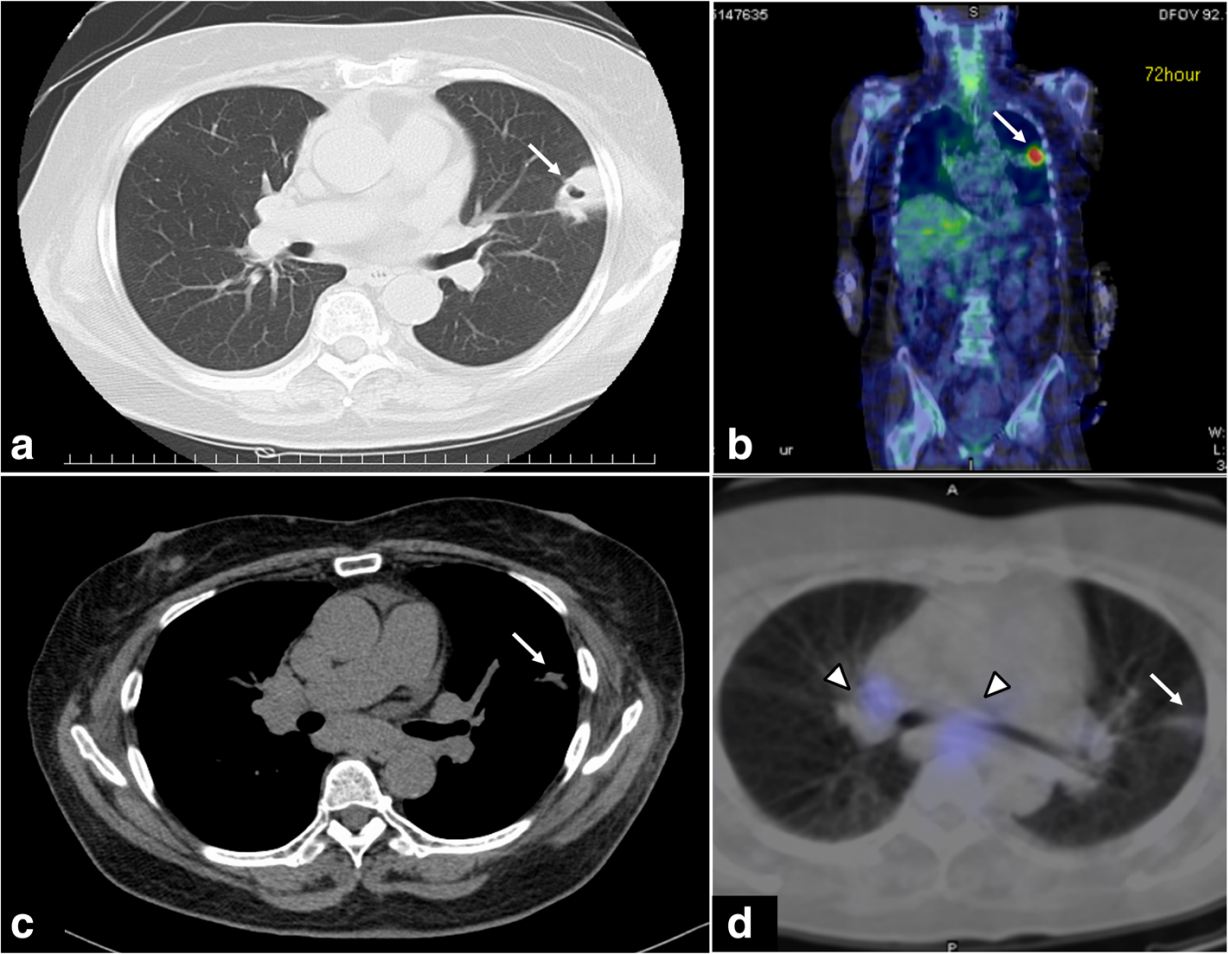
Imaging studies of the lung nodule at the time of hospitalisation (a, b) and 6 months after disease onset (c, d). a. Computed tomography (CT) at the time of hospitalisation. The white arrow indicates a nodule with a cavity in the upper lobe of the left lung. b. Gallium scintigraphy (^67^Ga-citrate) at the time of hospitalisation. The white arrow indicates high accumulation of gallium at the same location as the lung lesion. c. CT 6 months after disease onset. The lung lesion has disappeared, and a small scar remains. d. Somatostatin scintigraphy (^111^In-Pentetreotide) 6 months after disease onset. There is no accumulation in the left upper lobe (white arrow). The arrowhead indicates light accumulation of indium-111-radio-labelled octreotide in the mediastinum
